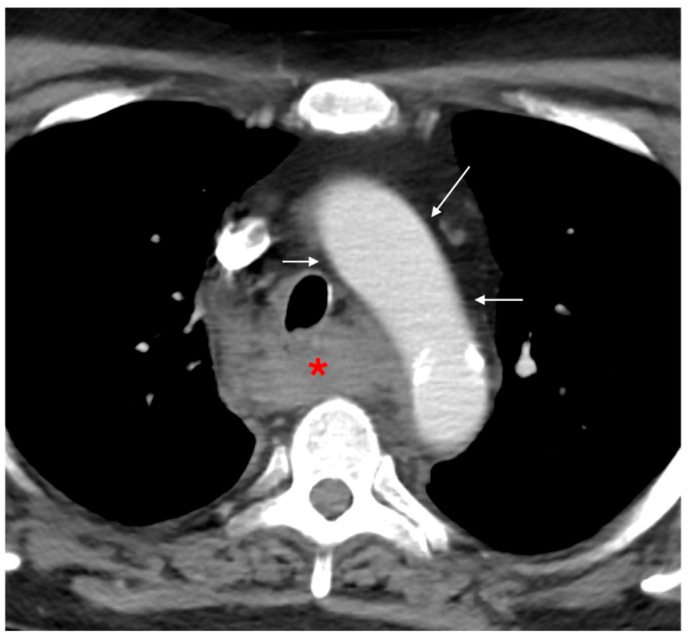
Mediastinal hematoma with preserved fat plane. Axial CTA image demonstrates a middle/visceral mediastinal paraesophageal hematoma (red asterisk) resulting from esophageal injury. The periaortic fat plane remains preserved (white arrows), without surrounding stranding, arguing against the aorta as the source of the mediastinal hematoma.
